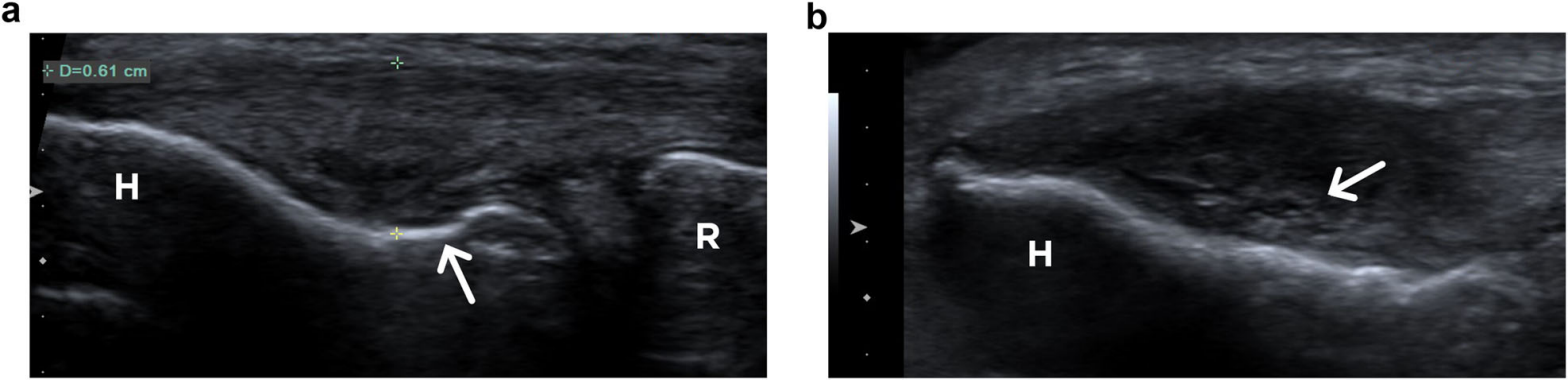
Ultrasound of the right elbow in a 49-year-old man with chronic lateral tendinopathy. a The thickness of the common extensor tendon and radial collateral ligament complex (between the cursors) is measured on a long-axis US, showing the minor groove (arrow) at the base of the lateral epicondyle. b The entire tendon was scanned to assess its echostructure. On a more anterior image, the tendon is thickened and hypoechoic and shows anechoic clefts (arrow) consistent with an echostructure grade 3. H, humeral epicondyle; R, radial head
